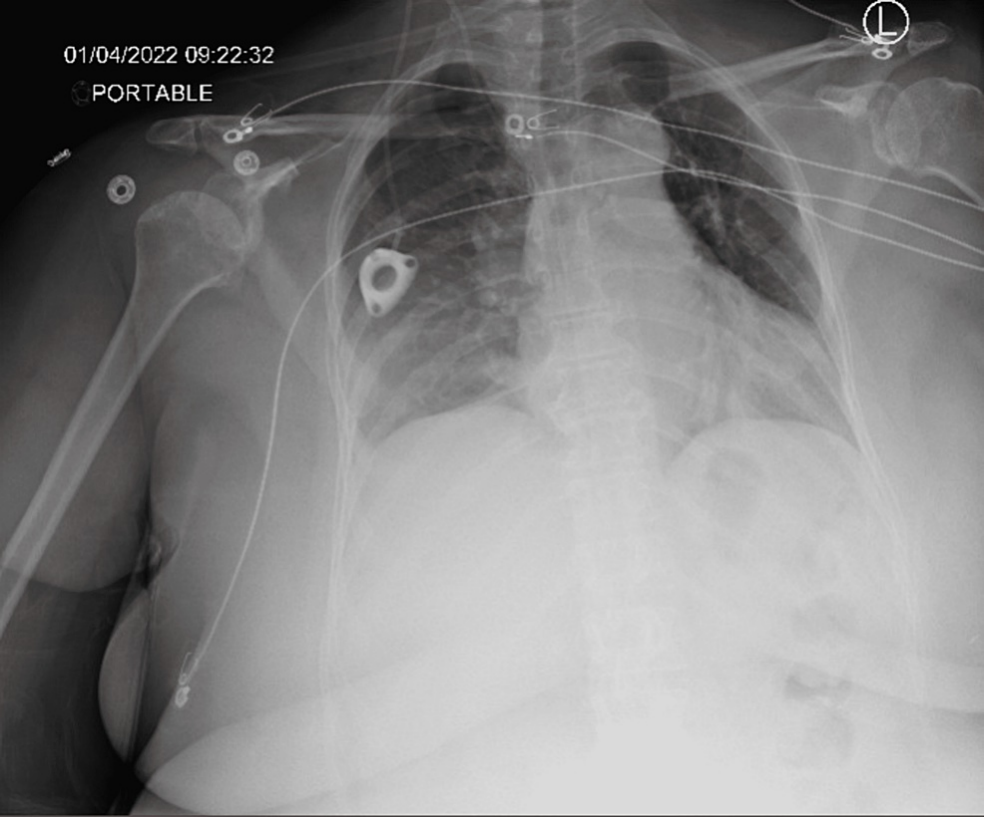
Initial postoperative chest radiograph The image shows a right internal jugular port in place with its catheter tip at the level of the cavoatrial junction. No pneumothorax or evidence of significant pleural effusion was noted.
